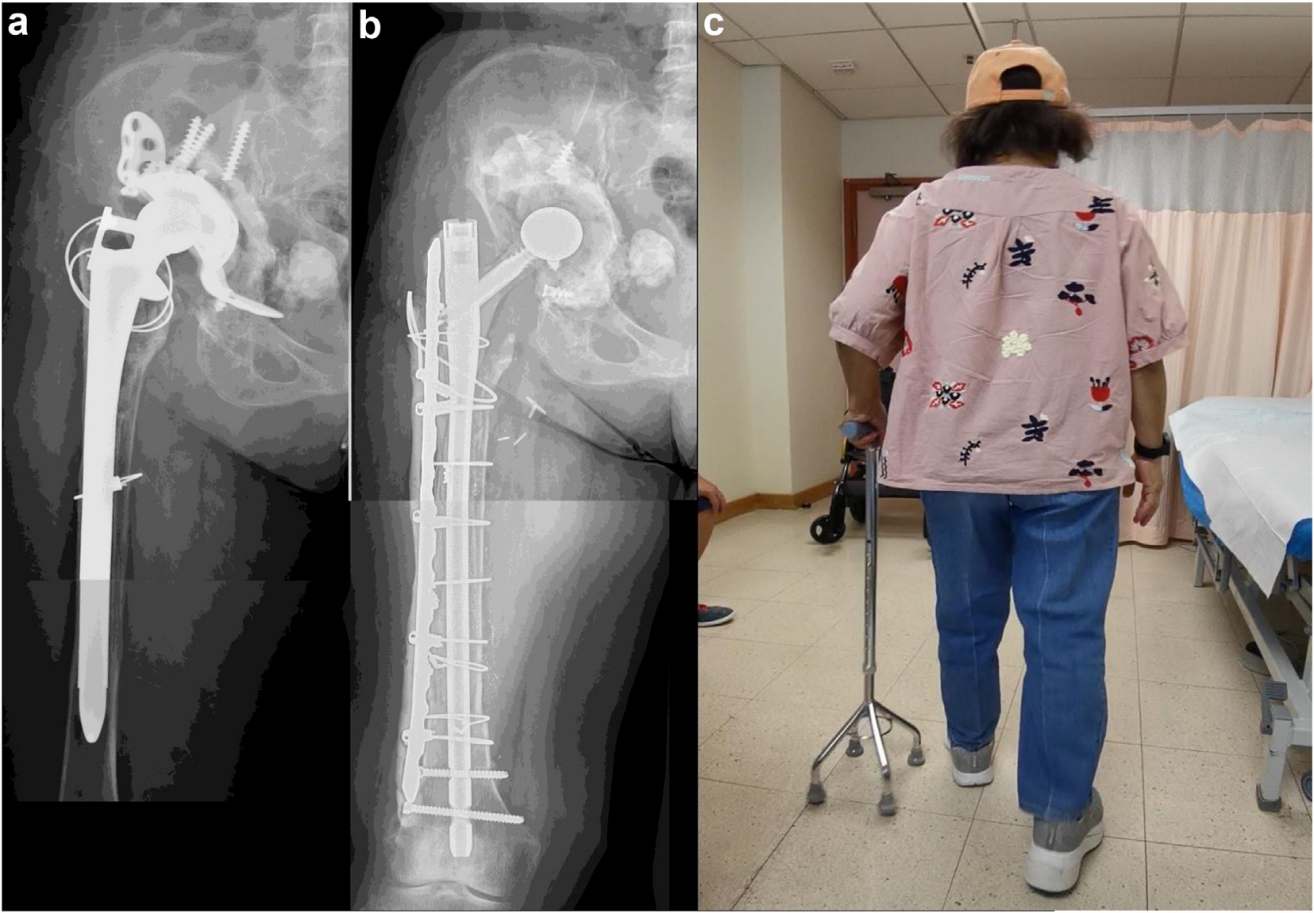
Case1 QMH. (a) AP radiographs demonstrating the failed revision THA with a long cylindrical porous-coated stem in situ. (b) AP radiographs after the first-stage reconstruction of the femur using a gamma nail and lateral femoral plate coated with antibiotic-loaded cement. (c) The patient ambulating in the clinic with a quadpod and a shoe raise 9 months after her first stage revision.
